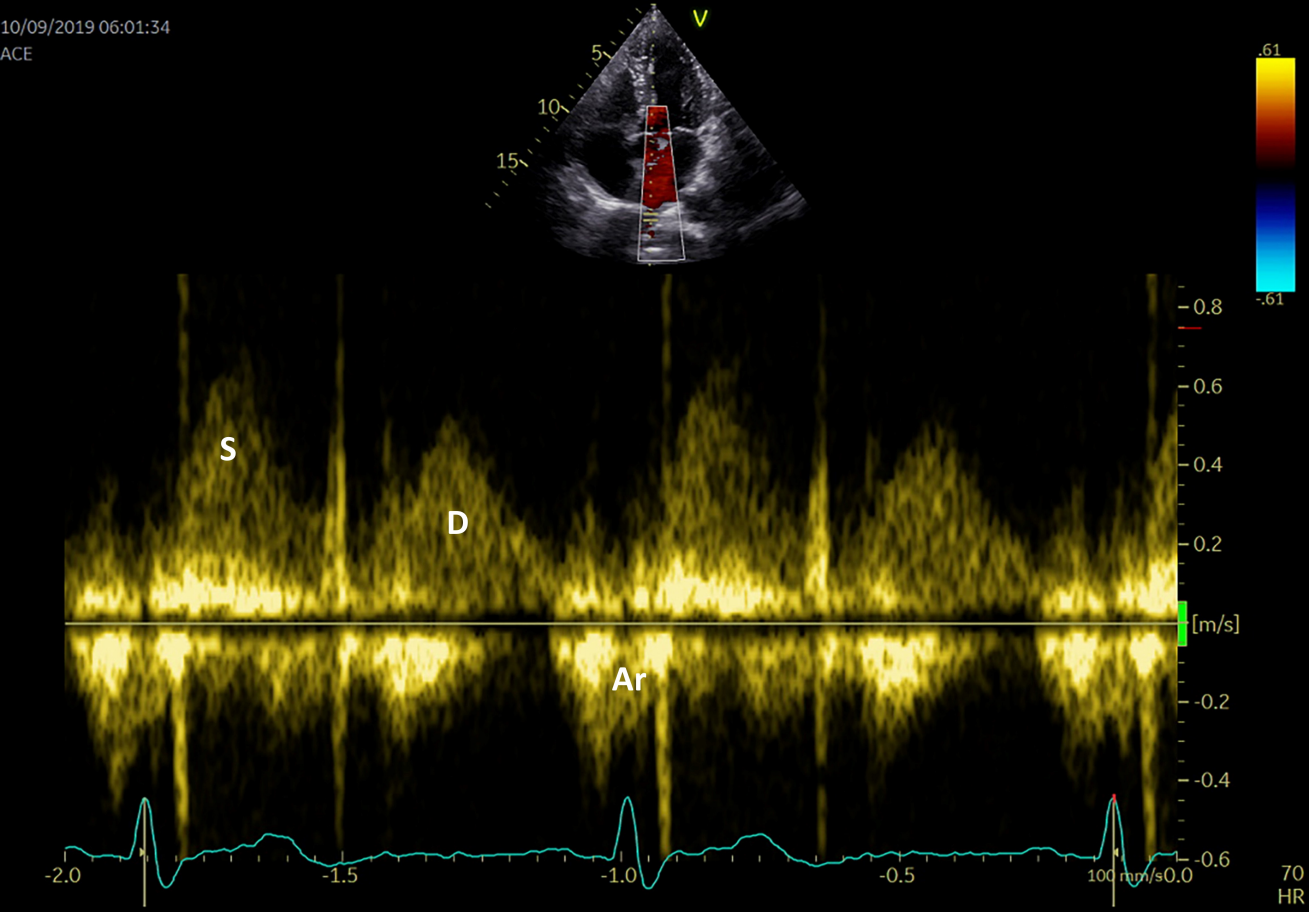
Pulmonary venous flow using PW Doppler echocardiography S
